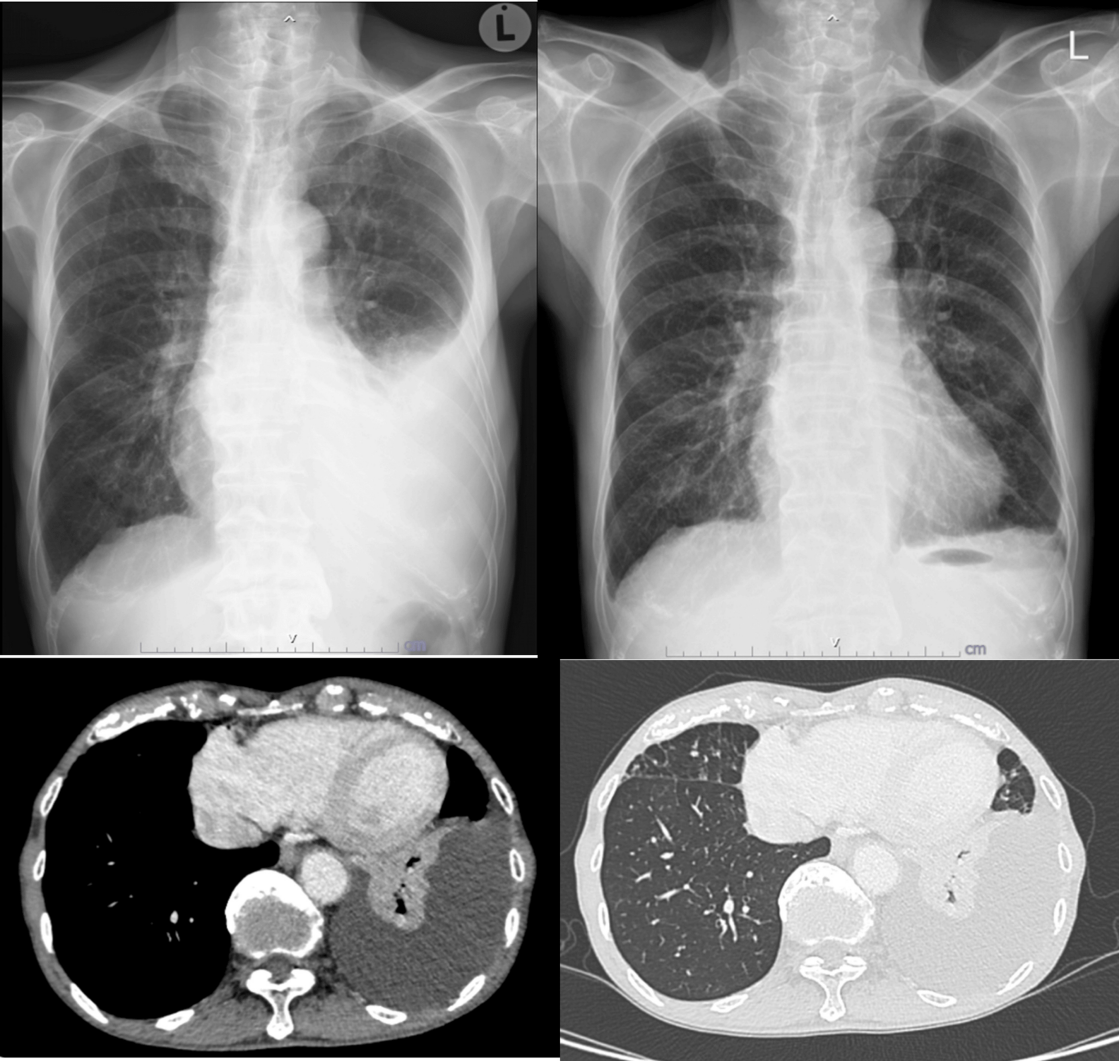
Left: Chest X-ray shows left pleural effusion. Right: Chest X-ray after two weeks of prednisolone treatment, demonstrating resolution of the effusion. The bottom row shows CT images revealing left pleural effusion without pleural thickening or significant mediastinal lymphadenopathy.
